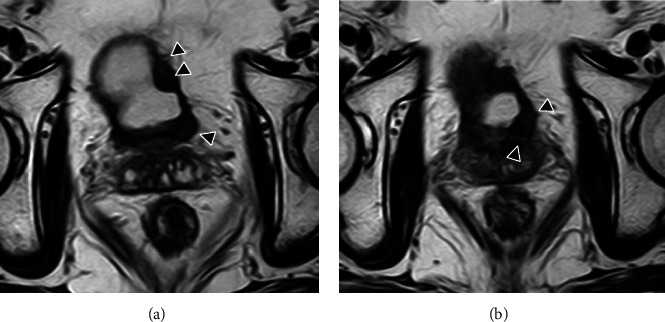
T2-weighted magnetic resonance image showing multiple recurrent tumors with muscle layer infiltration at the left laterally wall (a) and the neck (b) of the urinary bladder (arrows).
